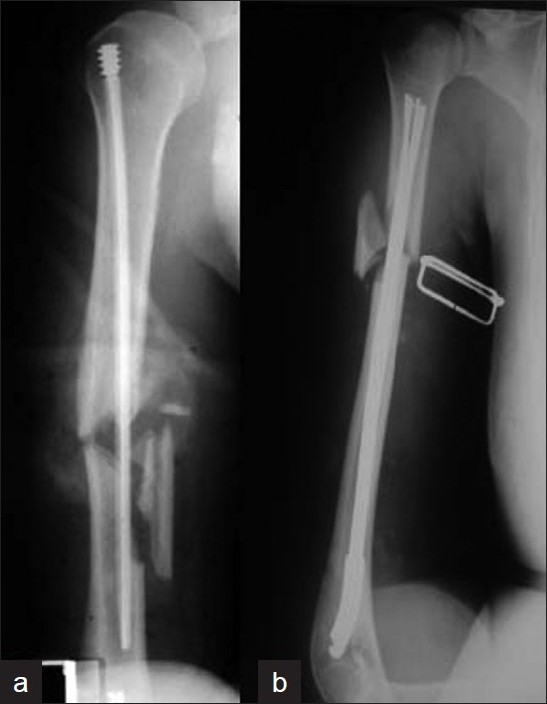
(a) X-ray of arm (anteroposterior view) depicting an example of inappropriate antegrade “Bio” nailing. This humeral nail (True-Flex, Encore) should be used in more proximal humeral fractures, as its locking facility depends on the nail-endosteum contact within the distal fragment. (b) Plain radiograph of arm (anteroposterior view) depicting inappropriate retrograde “Bio” nailing. This humeral nail (Marchetti- Vincenzi, Zimmer) should had been chosen longer in order to allow expansion of the rods within the humeral head
